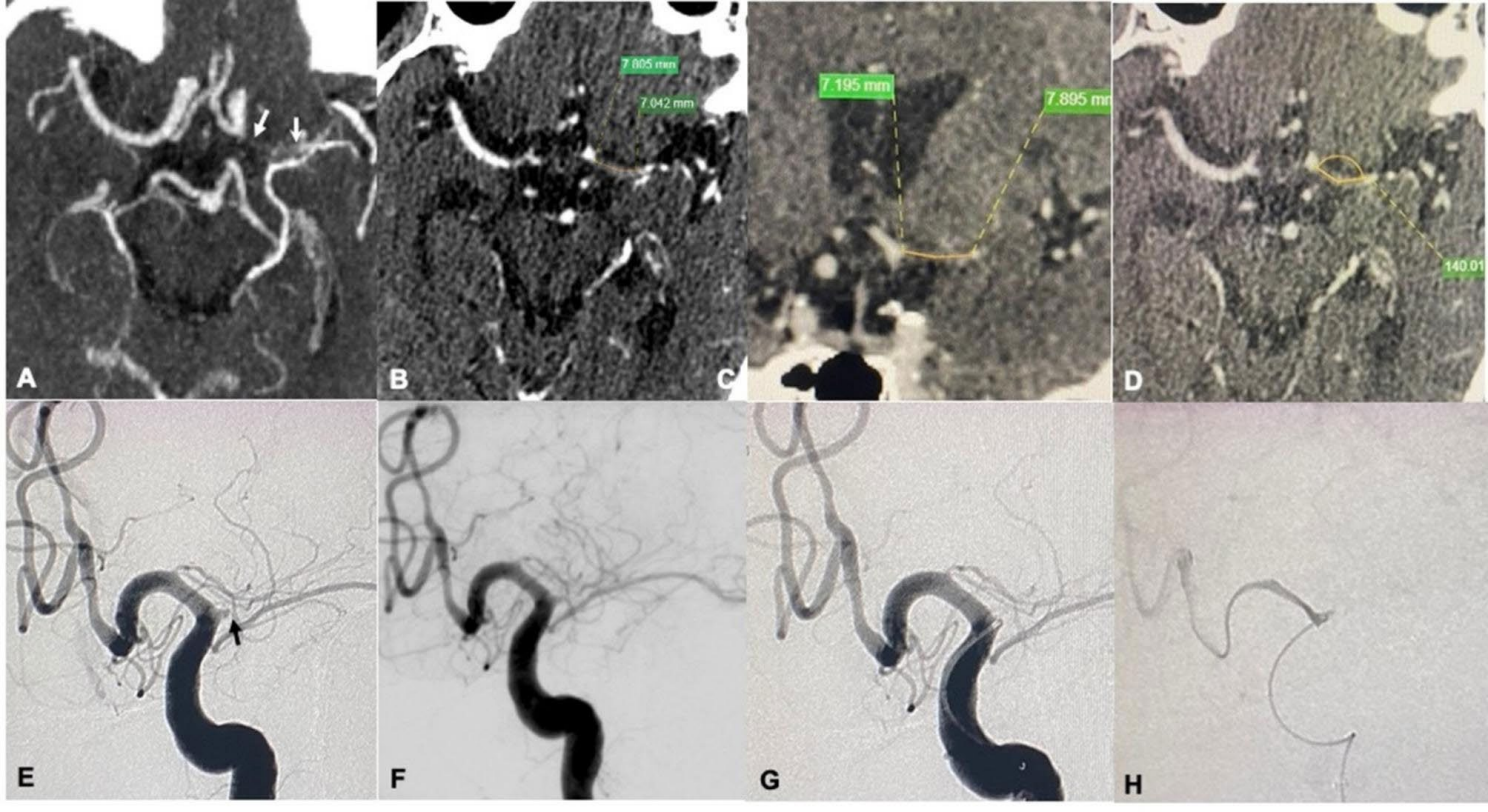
A patient with symptomatic middle cerebral artery occlusion (MCA) underwent endovascular recanalization attempts that were unsuccessful because the guidewire could not be advanced through the occlusion. A: Preprocedural computed tomography angiography (CTA) maximal intensity projection (MIP) images showed an occlusion at the origin of the left MCA and the proximal site (white arrow) and the distal site (white dovetail arrow) of the occluded segment. B and C: Multiple planar reconstruction (MPR) images of CTA images obtained from the axial (B) and oblique (C) views showed the total luminal filling defect at the occluded segment of the MCA. The occlusion length was approximately 15 mm. D: The occluded segment made a 40° turn from the proximal to the distal M1 segment. E and F: Digital subtraction angiography (DSA) confirmed occlusion of the left MCA origin (E), a short blunt stump of the occluded segment (white arrow), and no slow antegrade flow through the occluded segment on the mid-late arterial phase (F). G: The microwire and microcatheter were positioned to the origin of the left MCA, and attempts were made to pass through the occluded segment. H: After repeated attempts, the microwire and microcatheter could not cross the occluded segment, the injection by microcatheter showed no dissection and extravasation of contrast media, and the procedure was stopped
